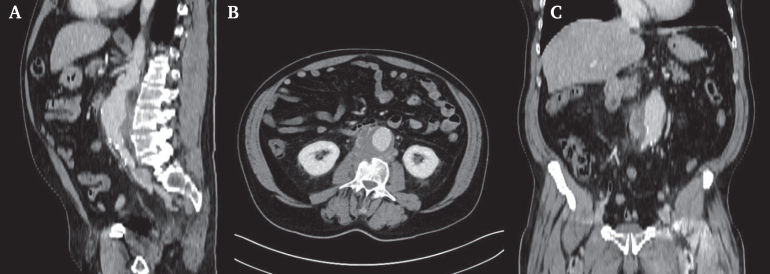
Pre-operative, contrast enhanced computed tomography showing in the (A) sagittal, (B) axial and (C) coronal planes, a juxtarenal aortic aneurysm with an oedematous fluid collection posteriorly.
